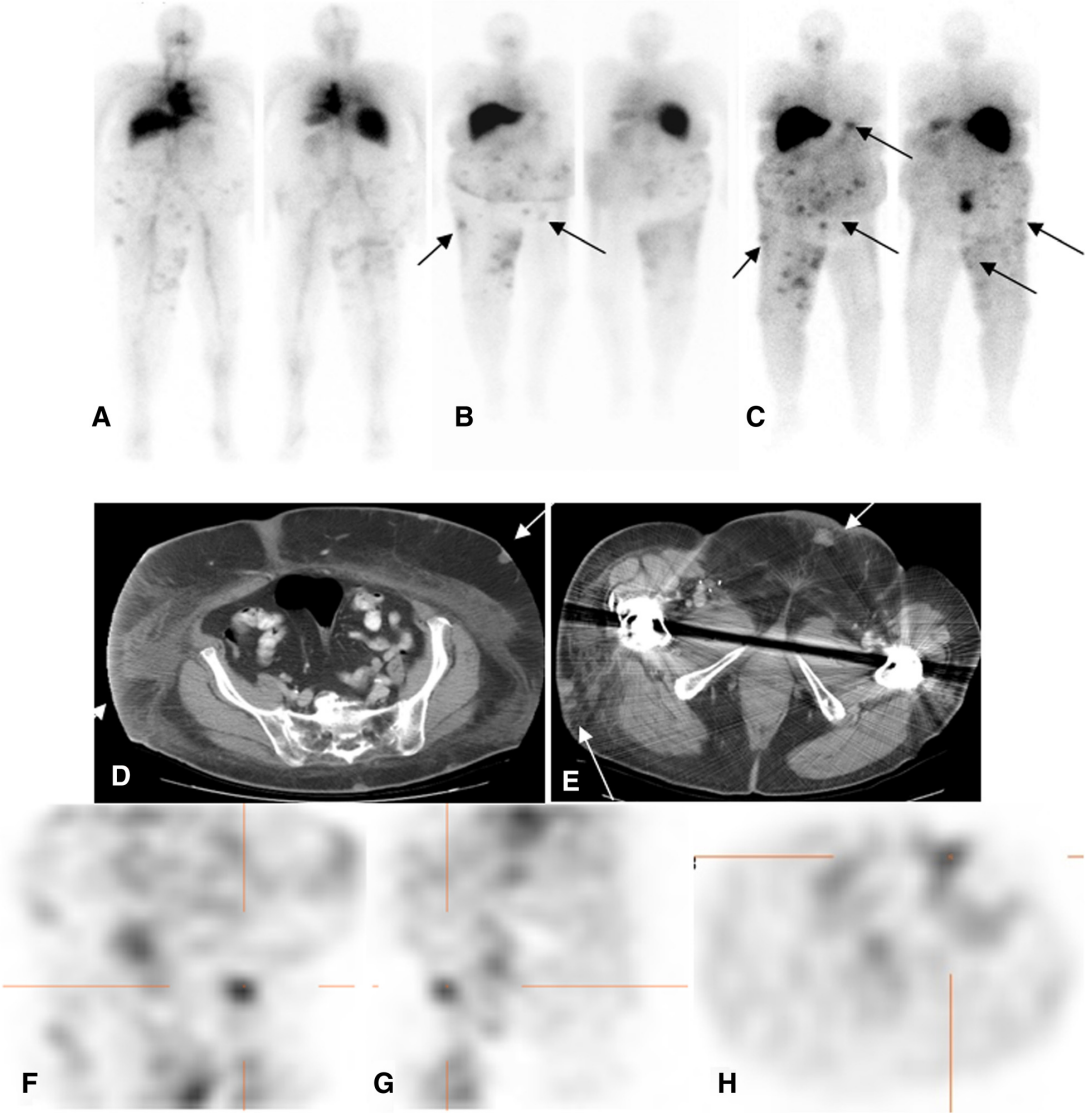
Patient with metastatic melanoma. Treated with 20 mg hu-J591 doses. Day 1, day 4, and day 7 images (A-C) after treatment cycle 2 show multiple foci of uptake in the skin and subcutaneous lesions (D, E) most prominent in the abdominal wall and right thigh. Uptake also localized focally on SPECT coronal, sagittal, and axial images (F-H) along known lesions.
